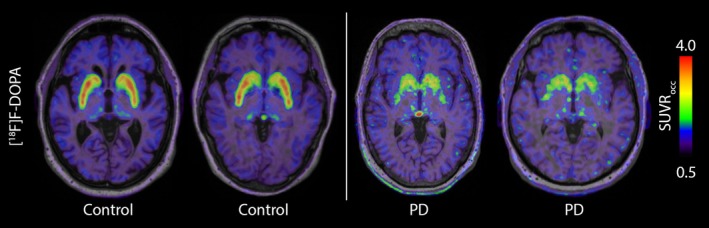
[^18^F]DOPA positron emission tomography (PET) in healthy controls and patients with Parkinson's disease (PD). Images of two healthy controls (left) and two participants with PD (right). Higher [^18^F]DOPA uptake is seen in the striatum of control participants, whereas uptake in PD patients is diminished reflecting the dopamine synaptic loss present in PD. Colour bar denotes standardised uptake value ratios (SUVRs) calculated using the occipital cortex (occ) as reference region where red equals higher [^18^F]DOPA uptake. Participants underwent [^18^F]DOPA PET as part of the Swedish BioFINDER studies (www.biofinder.se).
